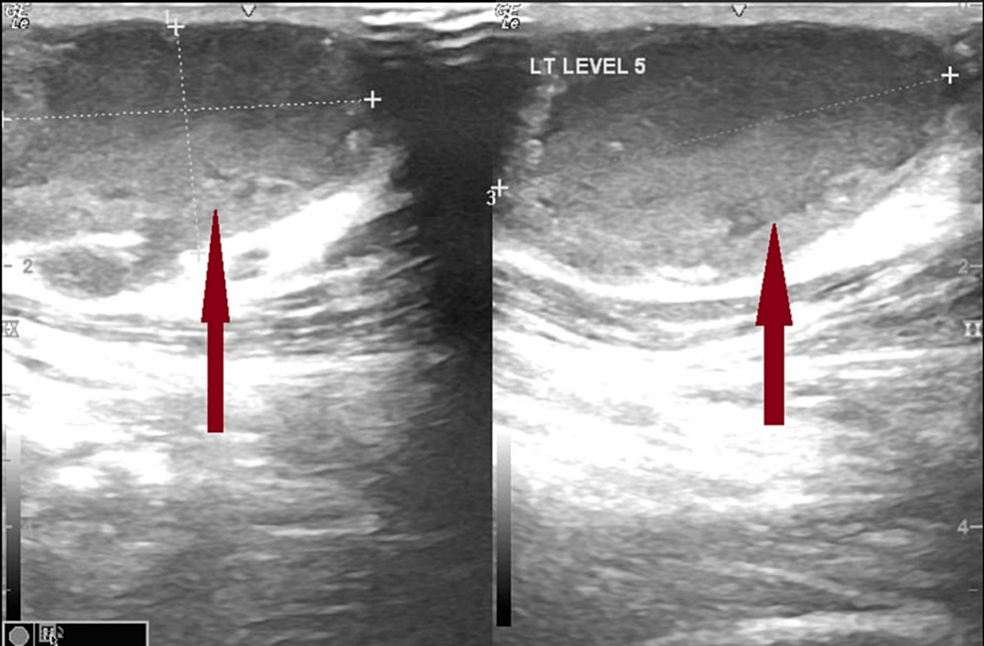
Ultrasound of neck showing bilateral conglomerated necrotic lymph nodes.
